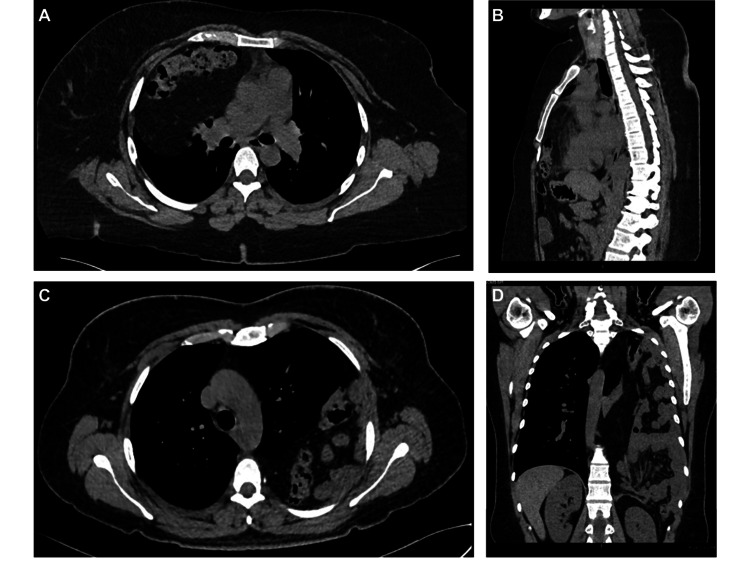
CT of a diaphragmatic hernia (A) Transverse image of a patient with a right antero-retrosternal (Morgagni) hernia. (B) Sagittal view of a patient with a right antero-retrosternal (Morgagni) hernia. (C) Transverse image of a left posterolateral (Bochdalek) diaphragmatic hernia. (D) Coronal view of a left posterolateral (Bochdalek) diaphragmatic hernia.
